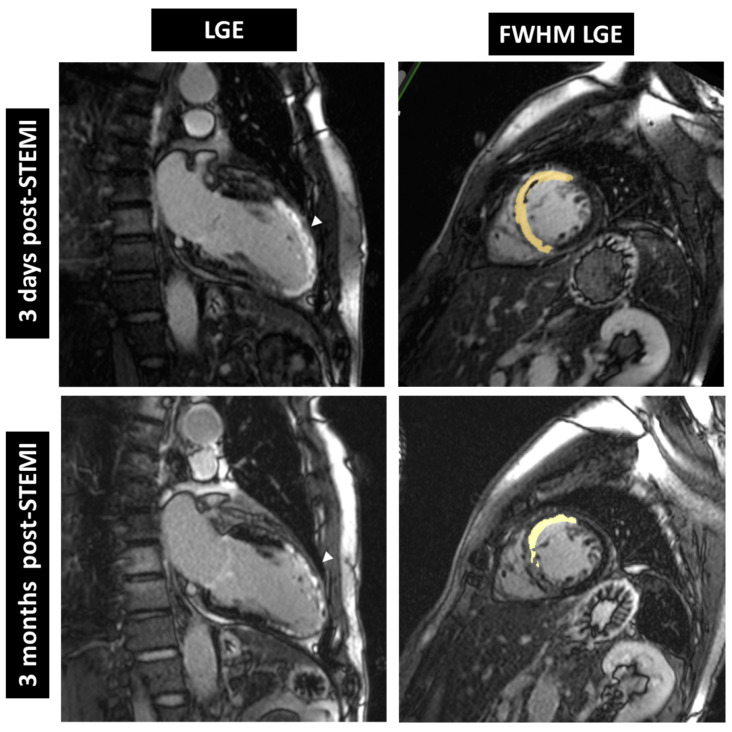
Cardiac magnetic resonance images from a patient receiving dutogliptin + filgrastim. LGE (left) and FWHM LGE (right) were reduced 3 months post-STEMI compared to baseline (3 days post-STEMI). LGE = late gadolinium enhancement, FWHM = full-width at half-maximum, arrow = LGE.
